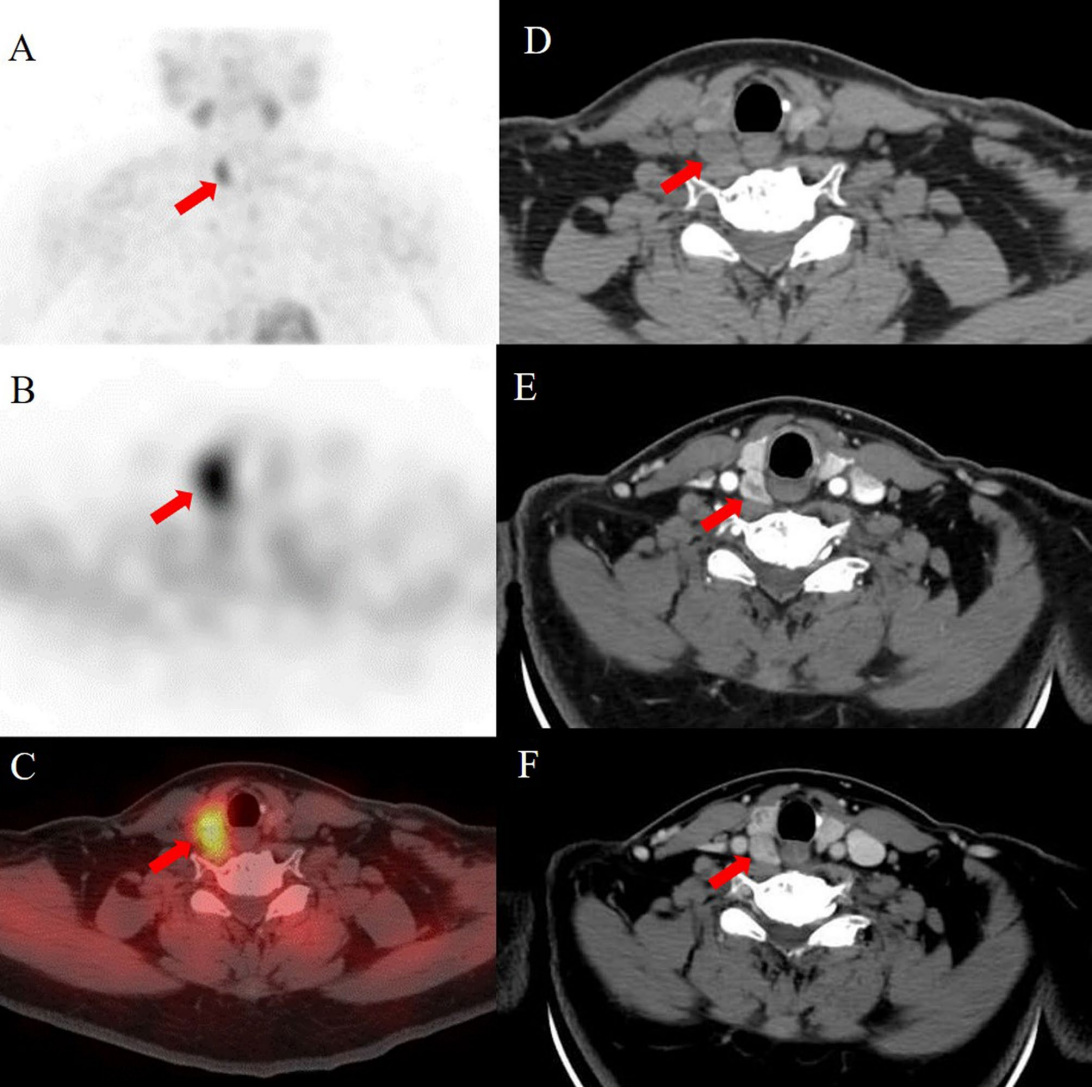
Images of a 53-year-old woman with primary hyperparathyroidism. Sestamibi SPECT/CT images (A, MIP SPECT; B, axial SPECT; C, fusion SPECT/CT) reveal focal radioactivity uptake localized to a single abnormal right superior parathyroid gland (red arrows). 4D-CT (D, axial noncontrast-enhanced; E, arterial phase; F, delayed phase) showing a vividly enhancing lesion at the arterial phase and washout of contrast material with decreasing attenuation compared with the atrial phase at the delayed phase (red arrows). The patient underwent parathyroidectomy for a single right superior parathyroid adenoma. Normal PTH and calcium levels were observed after 6 months of follow-up
